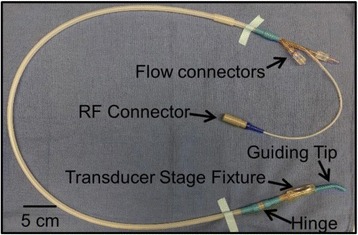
Prototype endoluminal ultrasound applicator assembly
